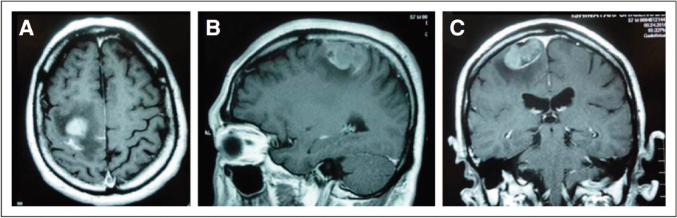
A brain magnetic resonance imaging examination (T1-weighted image) revealed a tumorous lesion (30 x 30 mm) showing a homogeneous and intense gadolinium enhancement in the right frontal to parietal lobe, surrounded by edema. Bleeding inside the tumor was also(Figure 6A, noted, axial; Figure 6B, sagittal; Figure 6C, coronal)^6)^
